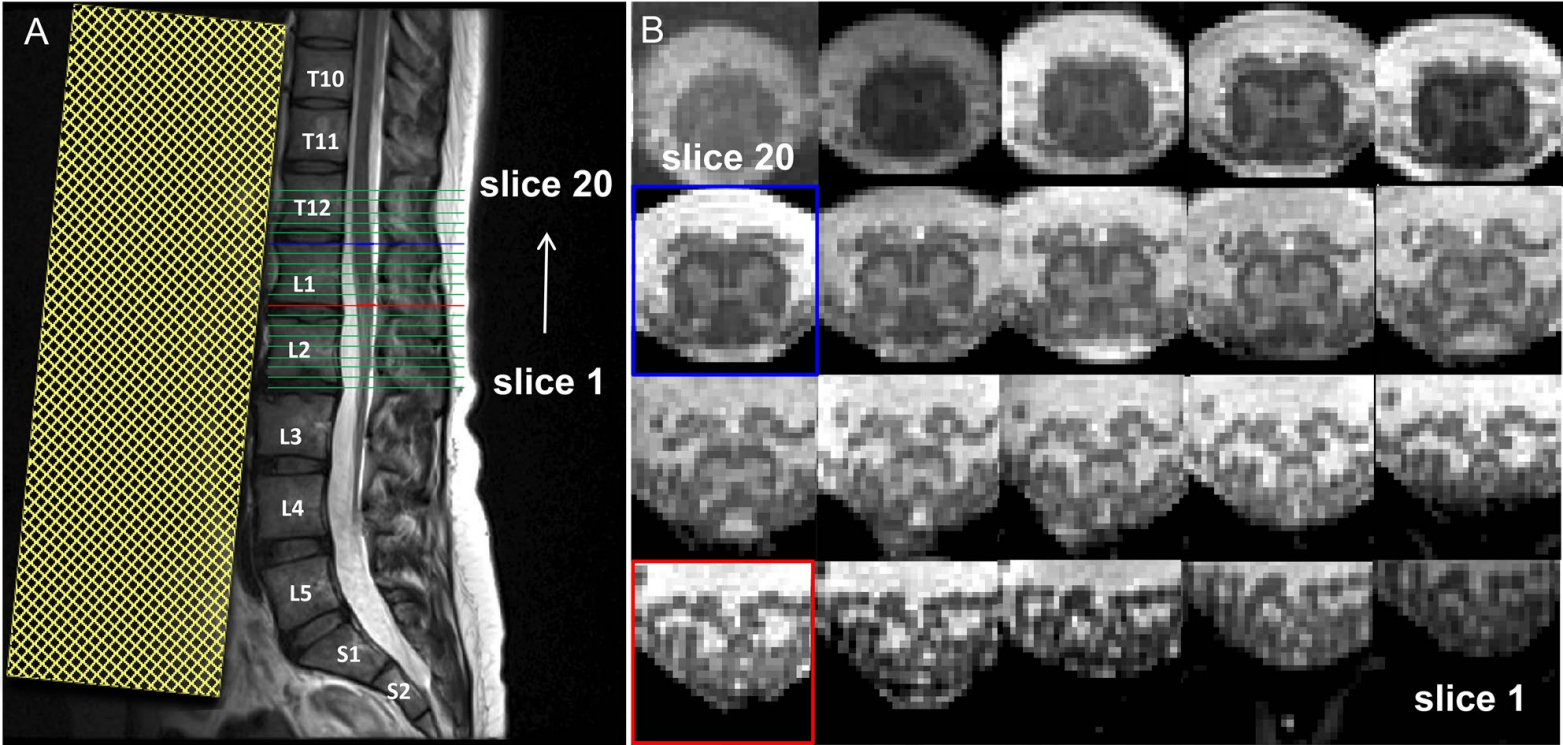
(A) Sagittal T2-weighted turbo spin echo acquisition in the lower spine used for subsequent prescription of the high-resolution axial acquisition. (B) Corresponding axial slices acquired with the 3D multi-echo gradient-echo sequence ( Siemens FLASH) in the caudal-rostral direction (slices 1–20). Highlighted are the slice in the lumbosacral enlargement (LSE) with the largest cord cross-sectional spinal cord area (defined as the "LSE slice" and shown in light blue in A and B; here: slice 15), and the most caudal slice in the conus medullaris (CM) where the gray matter still has the characteristic butterfly shape (defined as the "CM slice" and shown in red in A and B; here: slice 9). A saturation band, displayed as yellow shaded area in A, was placed anterior to the spine to suppress signal and possible artifacts arising from abdominal peristalsis.
